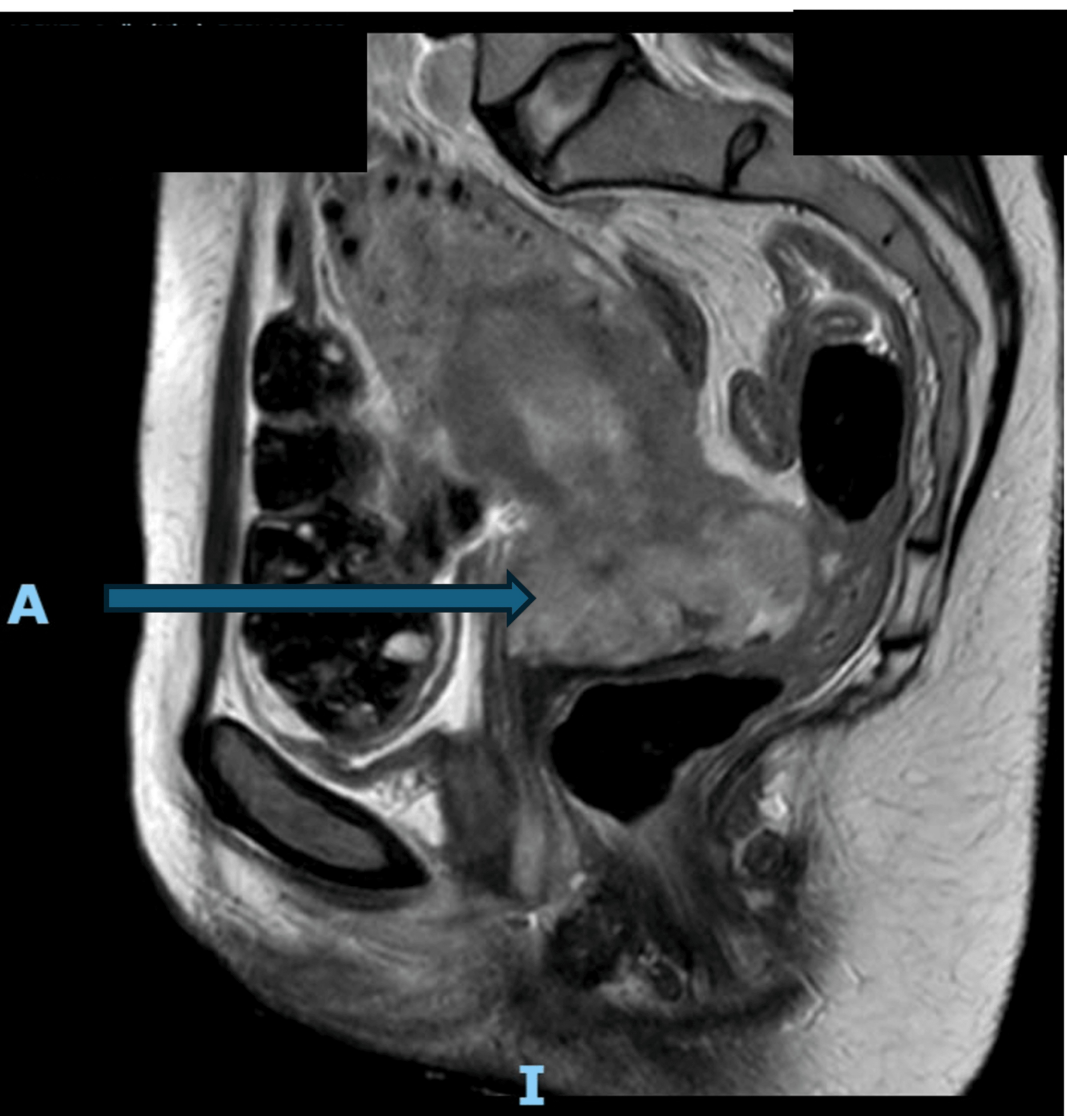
Sagittal T2-weighted pelvic MRI showing a bulky cervical tumor. Sagittal T2-weighted MRI demonstrates a heterogeneously hyperintense mass replacing the cervix (arrow). The lesion distorts the cervical anatomy and extends toward the lower uterine segment, with evidence of parametrial invasion. The rectum and bladder are compressed but not invaded.
